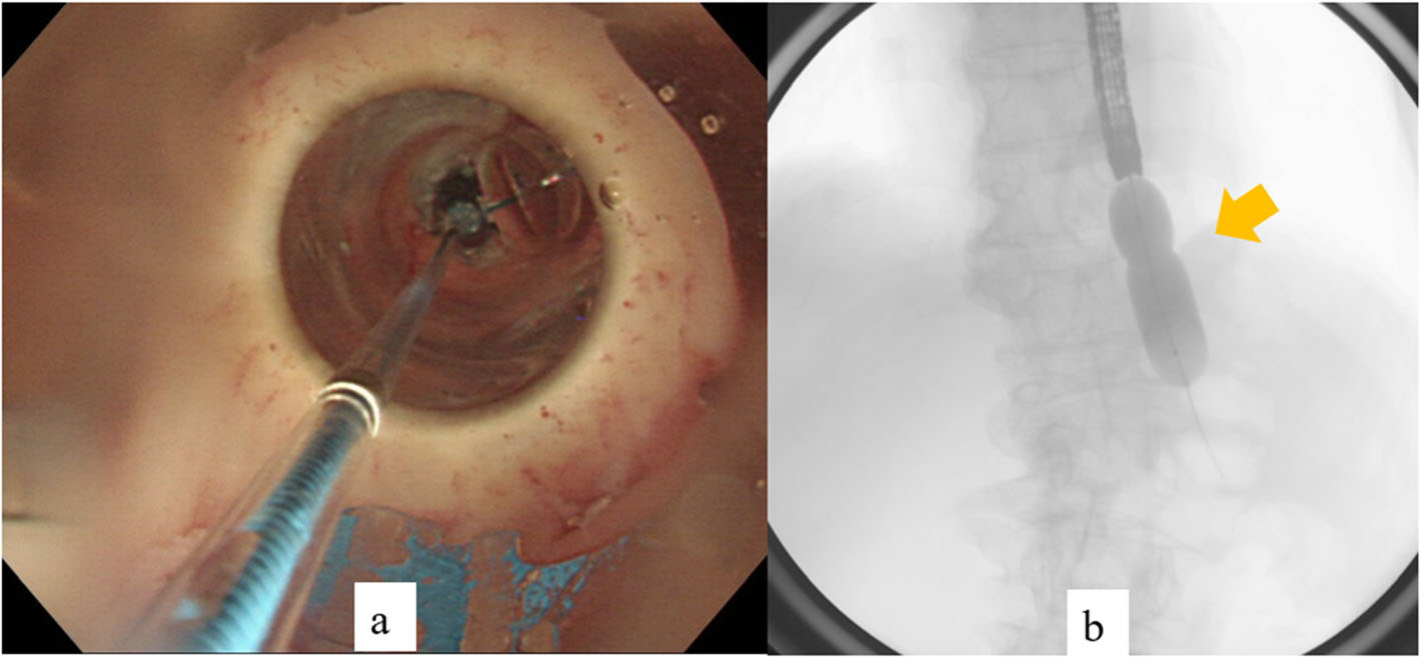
Endoscopic balloon dilation. a Esophagogastroduodenoscopy imaging. b Radiograph showing the “waist” caused by stenosis
